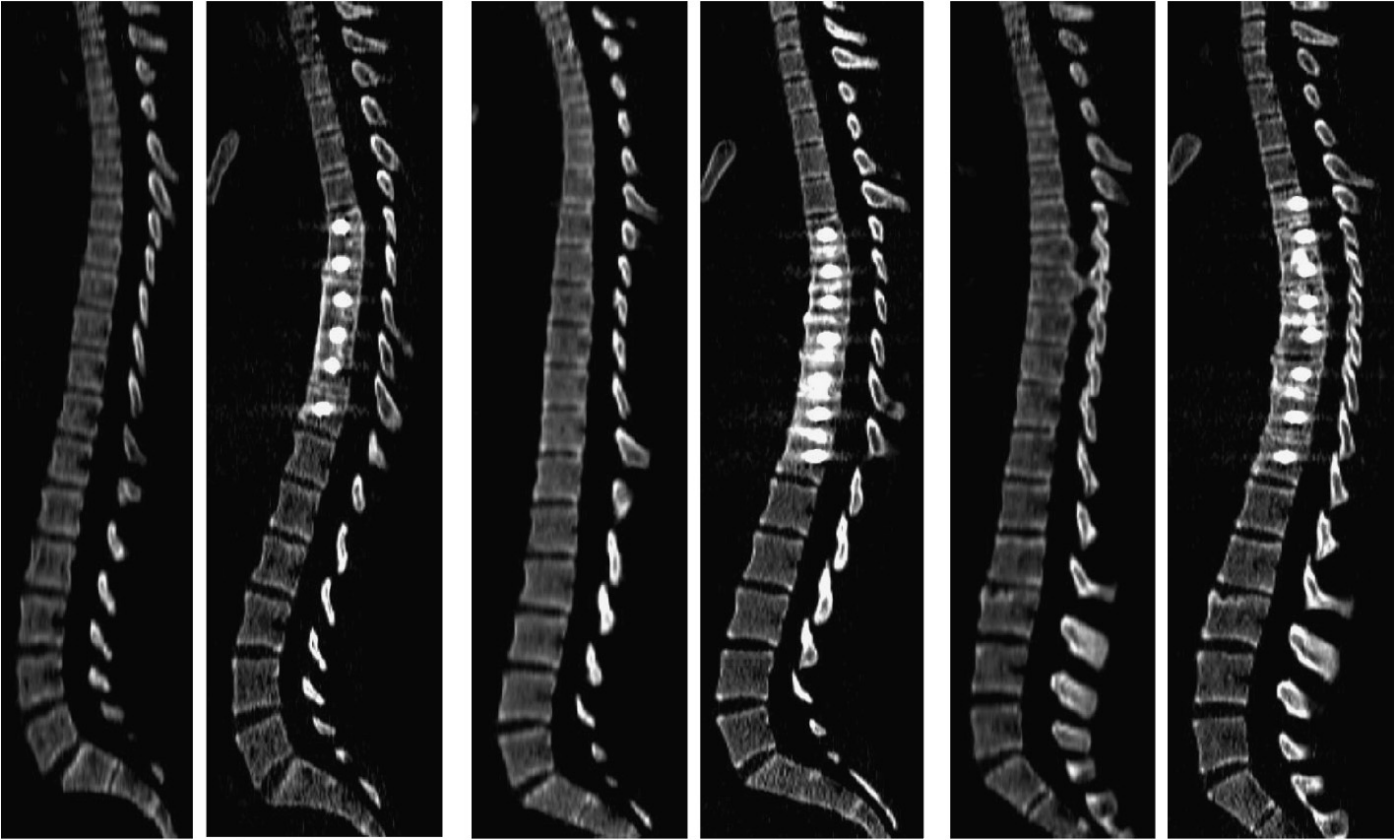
Resulting reformatted sagittal images for three patients in the study showing the paired sagittal CT reconstructions before and after surgery for each patient. Note the superior endplate definition compared to standing radiographs.
